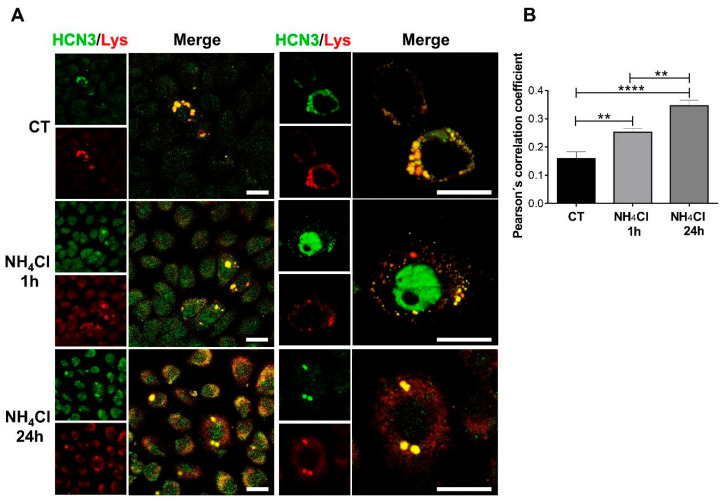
NH_4_Cl promotes time-dependent HCN3 expression in lysosomes (LysoHCN3). (A) Representative confocal images of NRK-52E cells in absence (CT) or presence of NH_4_Cl (1 and 24 h) with colabeling HCN3 channels (green) and lysosomes (Lys, red). Scale bar 20 μm. (B) Quantitative analysis of colocalization using Person’s coefficient. Data are represented as the mean ± SEM (*n* = 10 cells). Ordinary one-way ANOVA, followed by Newman–Keuls multiple comparisons post hoc test,  *p* < 0.01,  *p* < 0.0001.
